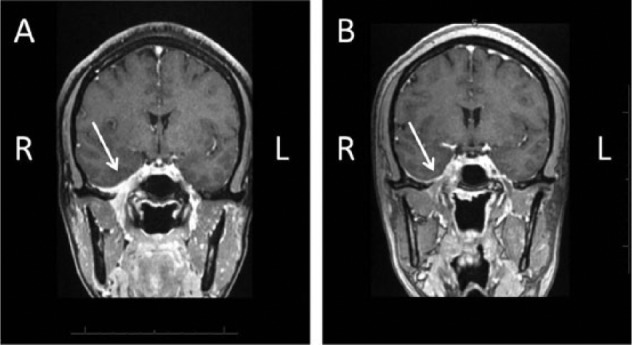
(A) MRI brain and orbits with and without contrast, coronal view, showing right-sided dural enhancement (arrow) of the middle cranial fossa, cavernous sinus, and foramen ovale. (B) MRI brain and orbits with and without contrast, coronal view, showing improvement in right-sided dural enhancement (arrow) of the middle cranial fossa after steroids.
